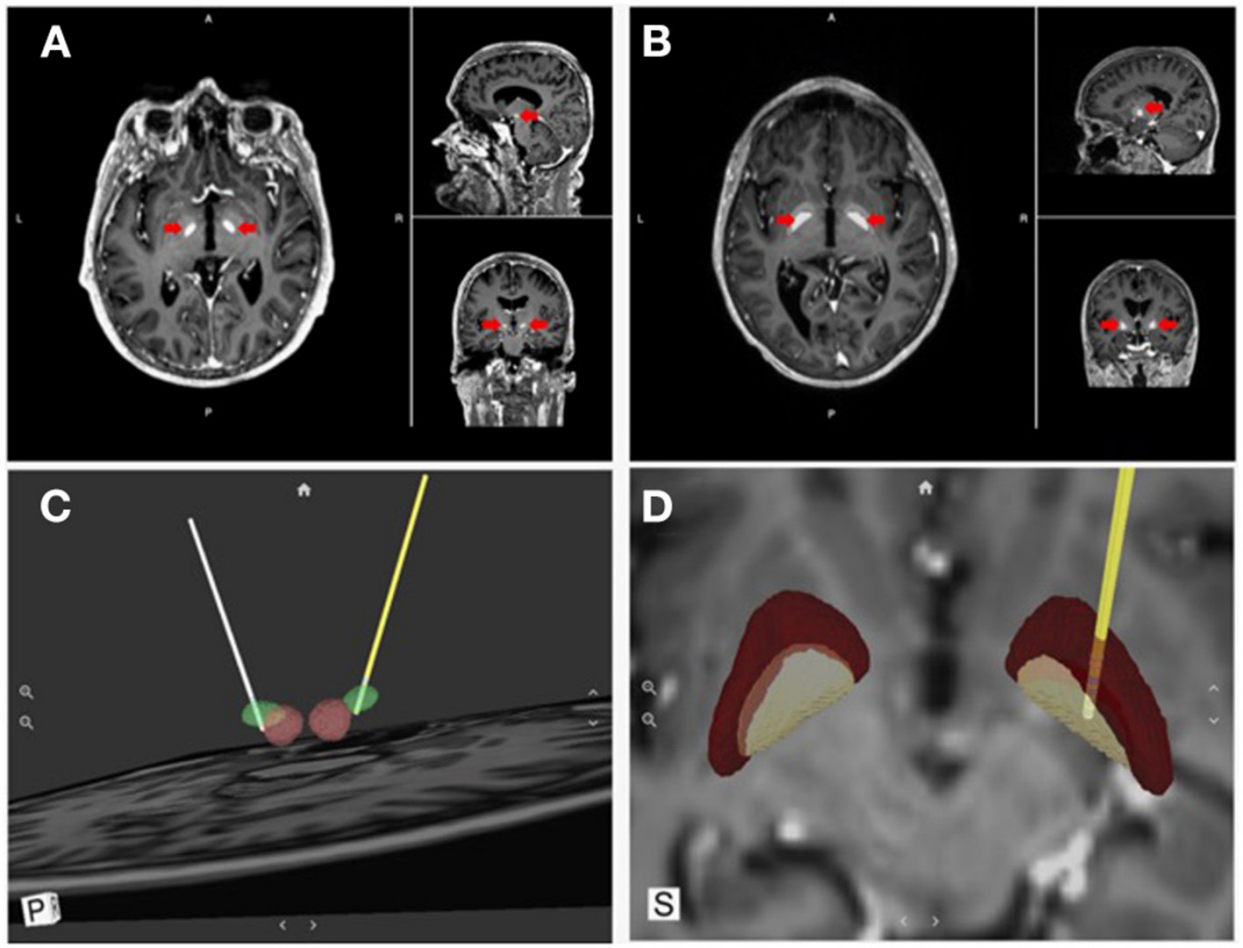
Direct visualization of DBS targets and lead location. Surgical Information Sciences (SIS), provides a patient-specific model of DBS targets using the patient's own enhanced clinical MR image. (A,B) shows alpha maps visualizing the STN and GPi, respectively (red arrows); (C,D) show 3D reconstructions of the intended targets registered with the DBS lead location in respect to the anatomical structures (STN & GPi, respectively).
